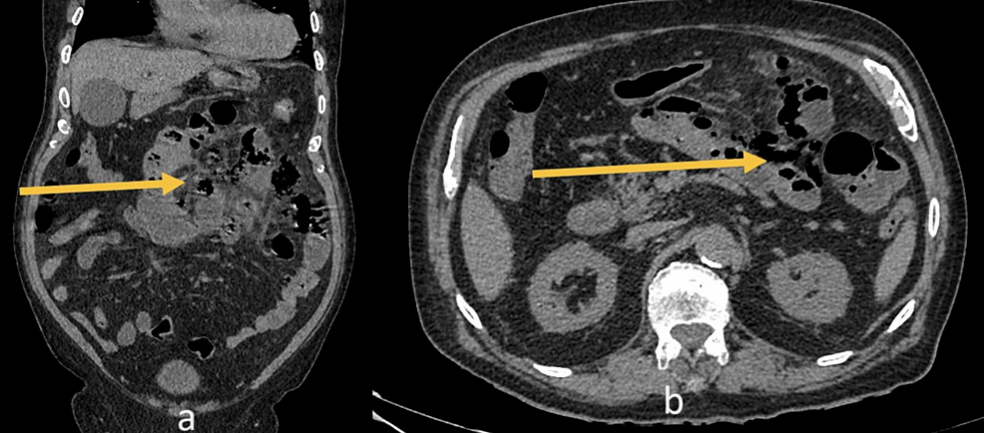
Contrast CT images of the abdomen and pelvis (a) coronal (b) cross-sectional views showing small bowel perforation with free air in the left upper quadrant (yellow arrows)
